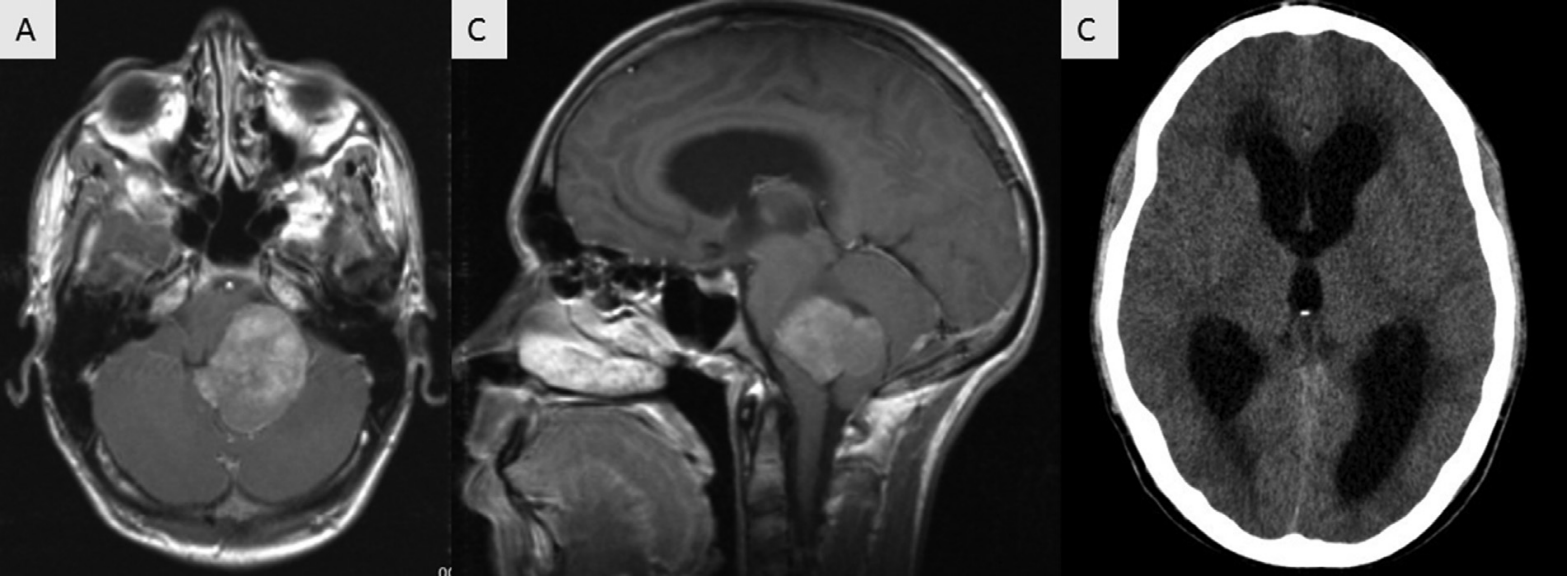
Preoperative magnetic resonance imaging (MRI) scans; axial (
